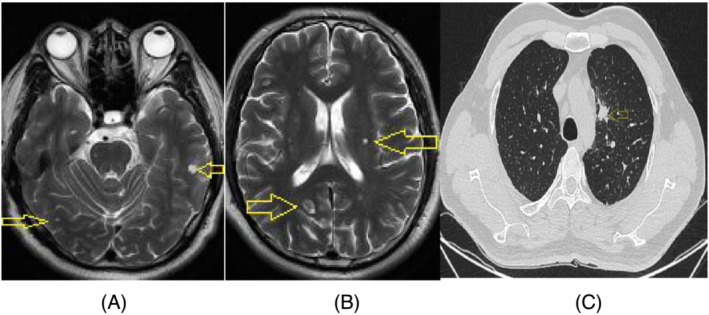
Magnetic Resonance Imaging (MRI) of brain and CT scan of lung on July 20, 2022. Seventeen months after Icotinib treatment, MRI of brain showed that multiple metastases (14 lesions) appeared in brain. But the bilateral choroidal lesions could not be determined by MRI. The CT scan of the lung showed the nodule in left upper lung did not significantly progress (C).
